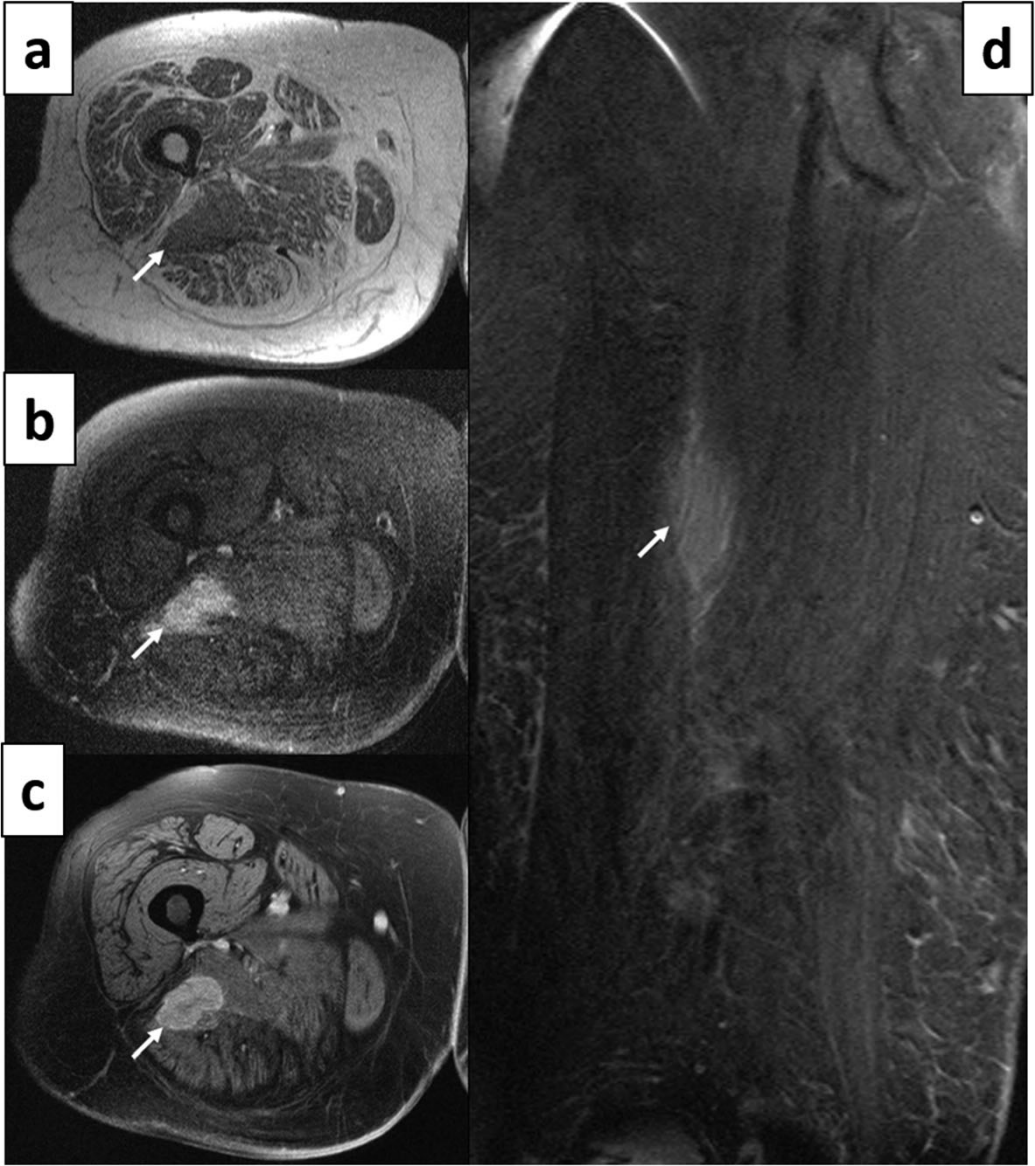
Case 3. 67 years old woman. 11 months post treatment follow-up MRI of the sciatic mass. a The axial T1 weighted image shows the hypointense mass within the right sciatic nerve (arrow) in the mid-thigh region. b The mass shows increased signal intensity in the fat-suppressed T2 weighted image and c post gadolinium enhancement in the fat- suppressed T1-weighted image (arrows in b and c). d Coronal STIR imaging demonstrating good response to treatment, with significant interval decrease in size of the tumor (arrow)
